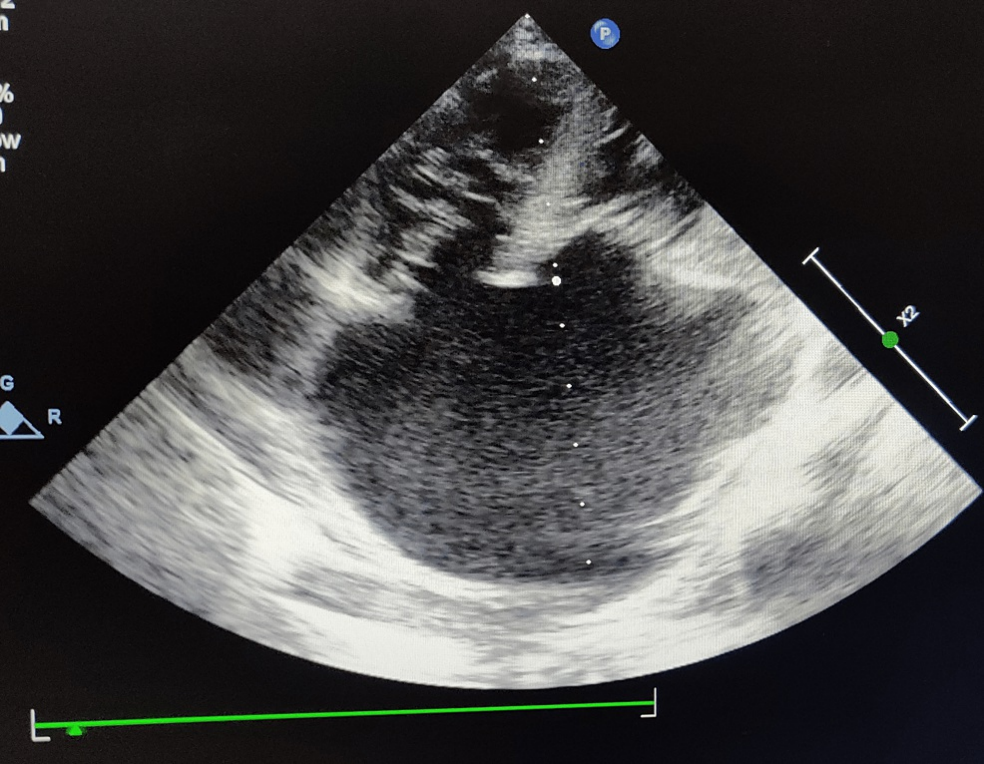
Common atrium and patent ductus arteriosus seen on echocardiography
